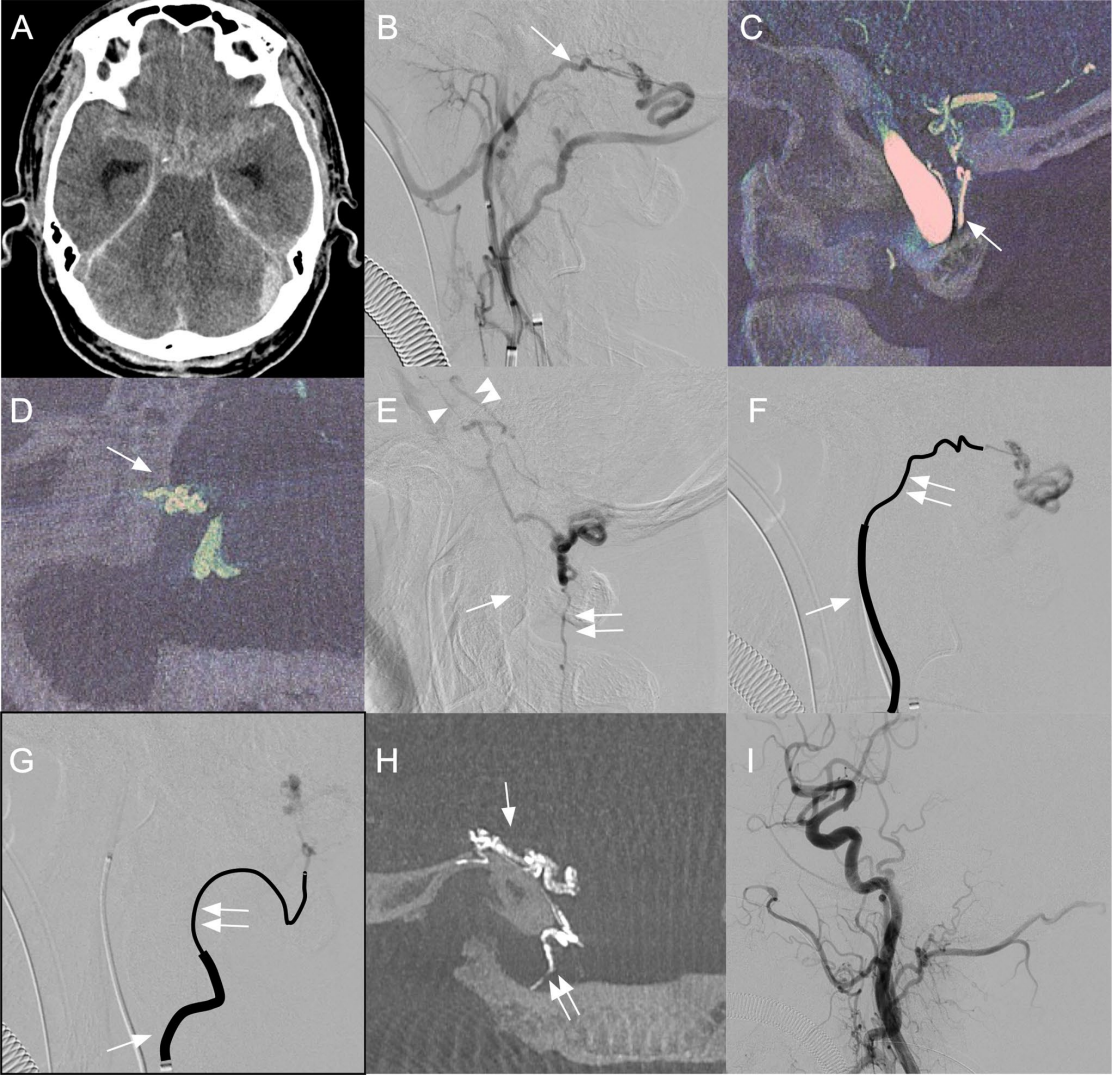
Representative case of the DAVF with fistulous point located on the lateral surface of the foramen magnum: MS-BV DAVF. (A) CT at admission showing subarachnoid hemorrhage. (B) Lateral view of the right external carotid angiography showing a DAVF at the level of foramen magnum. Arrow indicates jugular branch of the APA. (C) Sagittal MPR image of the right vertebral 3D angiography showing the C1 dural branch from VA (arrow) feeding the DAVF. (D) Axial MPR image of the right external carotid 3D angiography showing the feeder from the jugular branch continued to the fistulous points (arrow) on the lateral surface of the foramen magnum and then the medullary bridging vein. The DAVF was classified as MS-BV DAVF. (E) Late phase of the right external carotid angiography showing both cranial and caudal drainage routes from the MS-BV DAVF. Arrow, the anterior spinal vein; double arrows, the posterior spinal vein; arrowhead, the left lateral pontine vein; and double arrowheads, the right lateral pontine vein. (F) Lateral view of selective angiography with contrast injection from a microcatheter shows the tip located deeply in the feeder of the jugular branch of the right APA. Double arrows indicate a 1.3-Fr micro catheter which was coaxially introduced through a 2.7-Fr high-flow microcatheter (arrow). (G) Lateral view of selective angiography with contrast injection from another microcatheter located in the C1 dural branch of the right VA just before NBCA injection. Double arrows indicate a 1.3-Fr microcatheter which was advanced through a 3.4-Fr distal access catheter (arrow). (H) Coronal MPR image after NBCA injection from both feeders showing cast of the NBCA filling in the feeders and the drainage vein. Arrow shows the feeder from the jugular branch of the right APA and double arrow shows the C1 dural branch of the right VA. (I) Postoperative right common carotid angiography showing complete obliteration of the MS-BV DAVF. MS-BV DAVF = marginal sinus bridging vein dural arteriovenous fistula, MS = marginal sinus, BV = bridging vein, VA = vertebral artery, NBCA = n-butyl-2-cyanoacrylate
